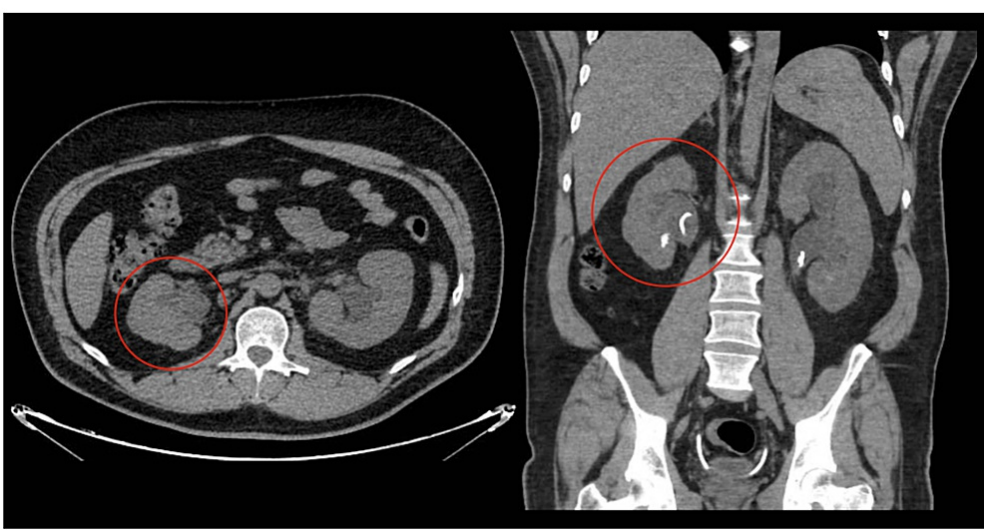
Axial and coronal views of the admission non-contrast CT The red circles show an atrophic right kidney with hydronephrosis and a double-J ureteral stent in place. A single non-obstructing stone is seen on the lower pole of the right kidney. CT: computed tomography
